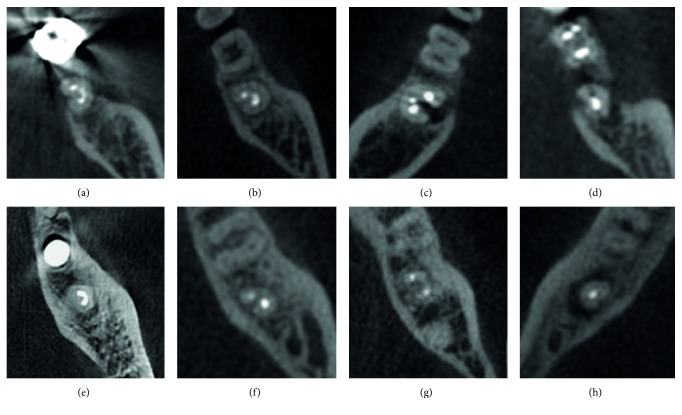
Classification of C-shaped canal configuration using cone-beam computed tomography. (a) C1 configuration at coronal level. (b) C2 configuration at coronal level. (c) C3 configuration at coronal level. (d) C4 configuration at coronal level. (e) C1 configuration at apical 3 mm level. (f) C2 configuration at apical 3 mm level. (g) C3 configuration at apical 3 mm level. (h) C4 configuration at apical 3 mm level.
